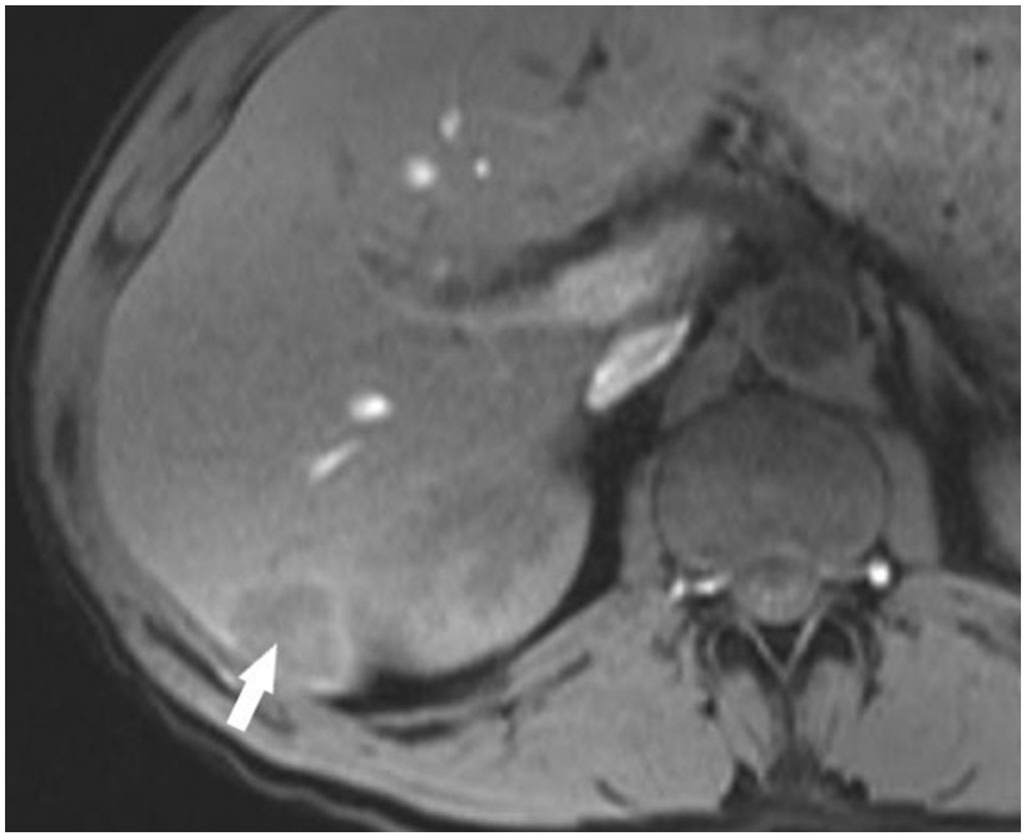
A hypovascular metastatic lesion in the liver. Liver MRI TI W image on post-contrast arterial phase showing a hypo-intense lesion with subtle concentric perilesional enhancement in segment VI of the liver (arrow).
